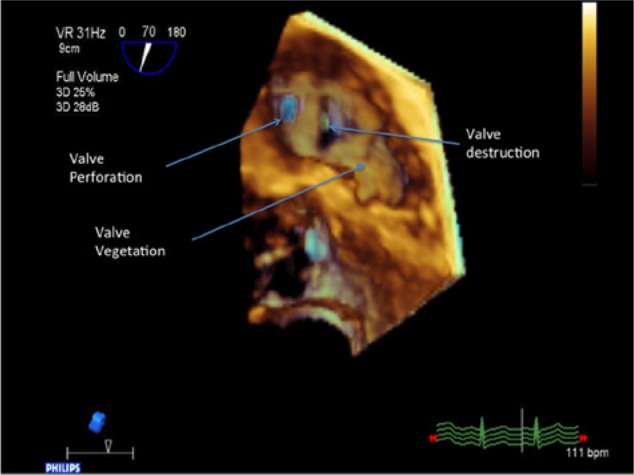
Transesophageal echocardiogram with 3D pulmonary valve with multiple valve perforation visible.
